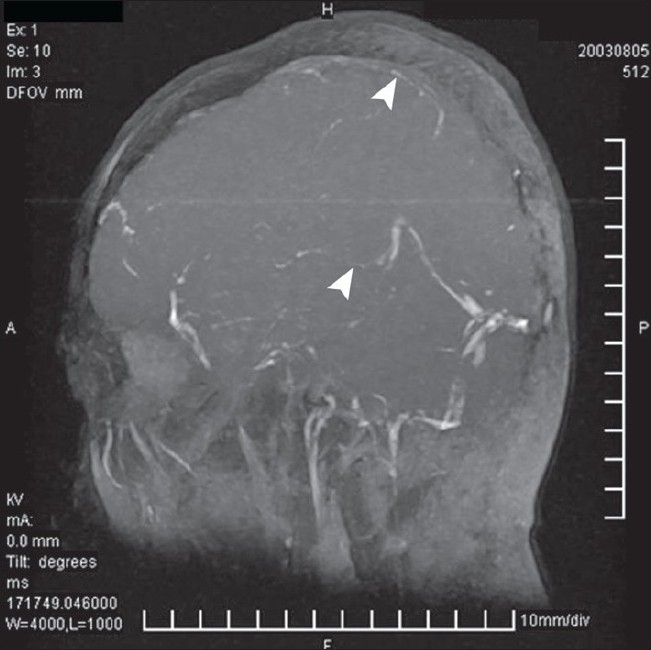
Magnetic resonance imaging showing flow signal void along the saggital sinus and transverse sinus, diagnostic of venous sinus thrombosis
